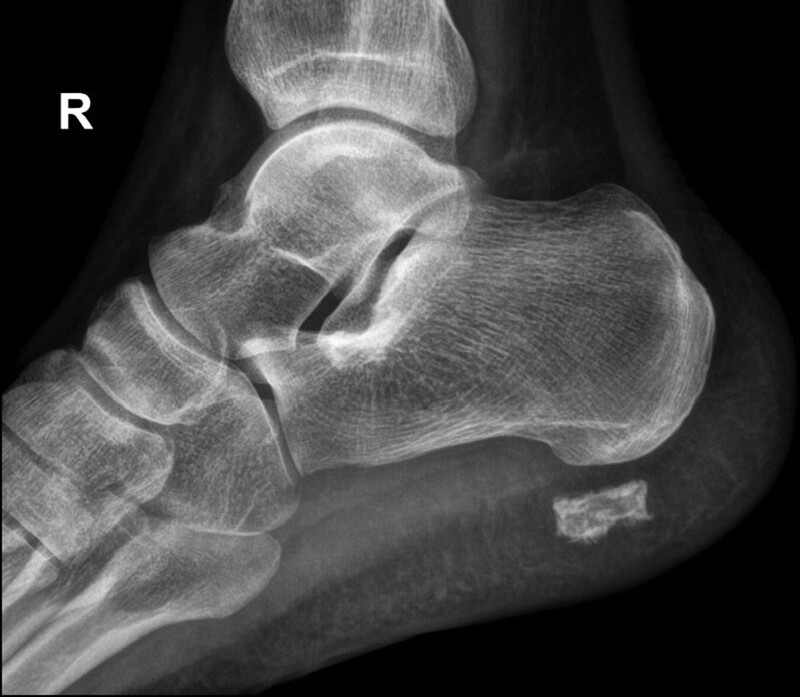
Right heel X-ray showing a patchy high-density shadow in the soft tissue of the lower edge of the right heel.
